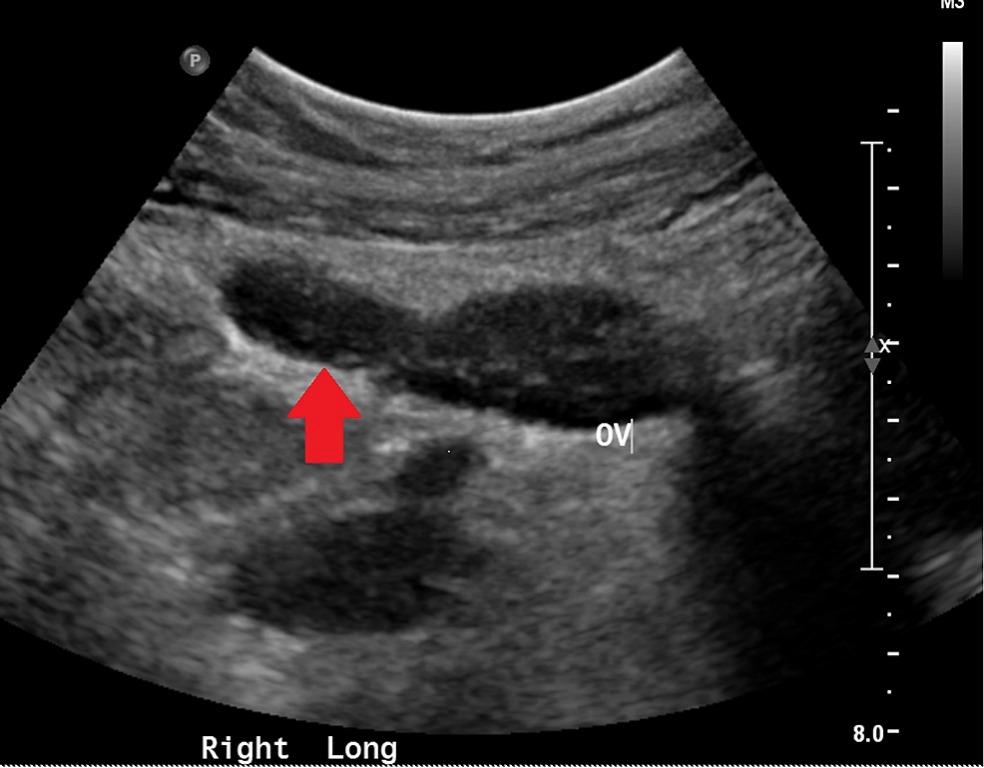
Ultrasound (US) showing bilateral dilated thick-walled fallopian tubes containing debris
